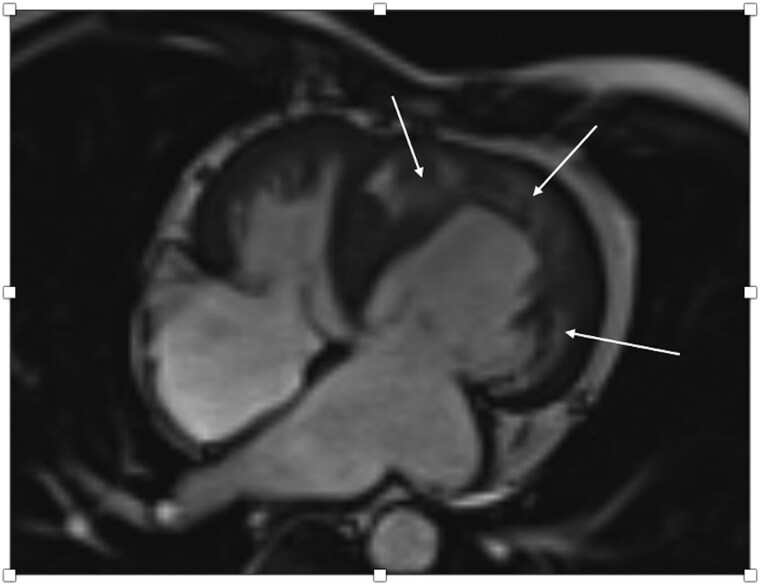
Cardiac MRI of a 63-year-old male with ACS after PCI showing impaired sRV function as well as subendocardial and transmural oedema in the sRV wall as well as the interventricular septum (arrows) correlating with an acute NSTEMI due to severe stenosis of the middle RCA and a CTO of both the left circumflex artery and the distal RCA.
